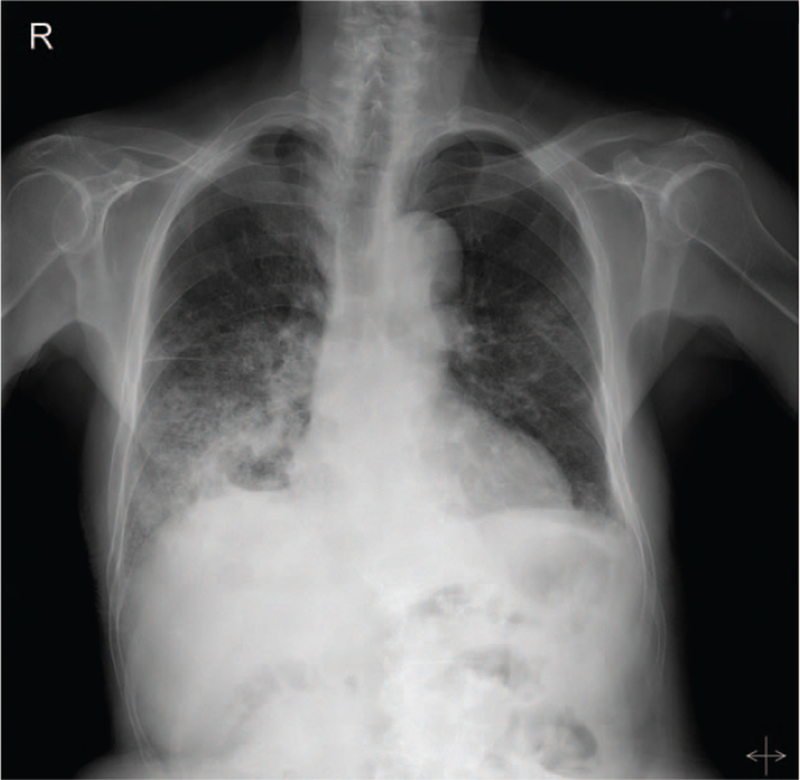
Chest radiography indicates increased opacities in the right lower lung field with an air-fluid level.
